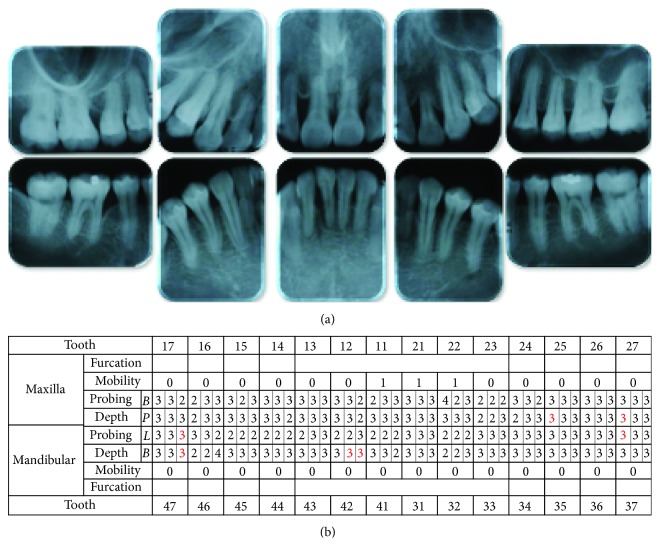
Post-orthodontic treatment dental radiographs (a) and periodontal examinations (b) are shown. The red color indicates bleeding on probing.
